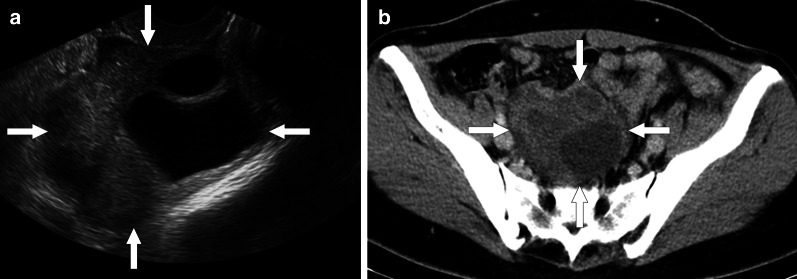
Chronic ovarian torsion. A 42-year-old female patient with no significant past medical history presenting with progressively increasing blunt pelvic pain in the last two months. a US study demonstrated 6 cm mostly solid appearing mass (with central and peripheral cystic areas) (arrows) within the right adnexa. Axial plane postcontrast CT scan b confirmed the presence of the sonographically detected mass (arrows). Postsurgical pathological examination showed no evidence of malignant disease but confirmed chronic ovarian torsion
